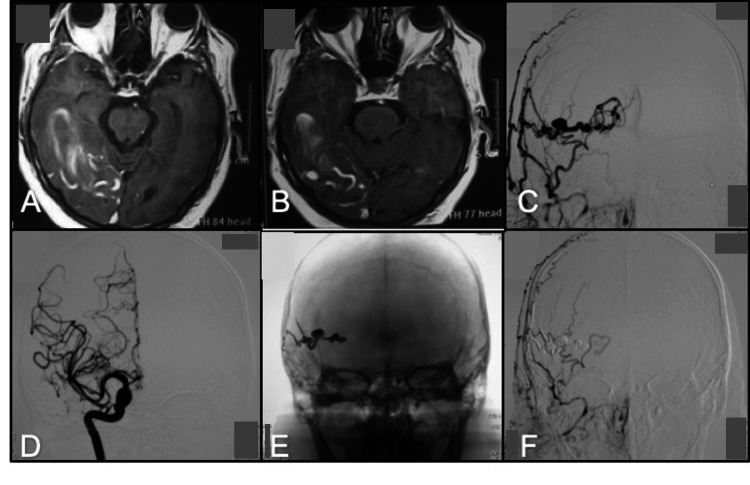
MRI axial T1 (A, B) Abnormal dilated vessels and hemorrhage; (C ) Anterior-posterior view, right ECA angiogram shows feeding arteries from the superficial temporal artery and occipital artery; (D) Right ICA angiogram shows no feeding arteries; (E) Fluoroscopic image depicting the final cast distribution of Onyx-18; (F) Right external carotid artery angiogram after embolization of the fistula with complete occlusion. ECA: external carotid artery; ICA; internal carotid artery
